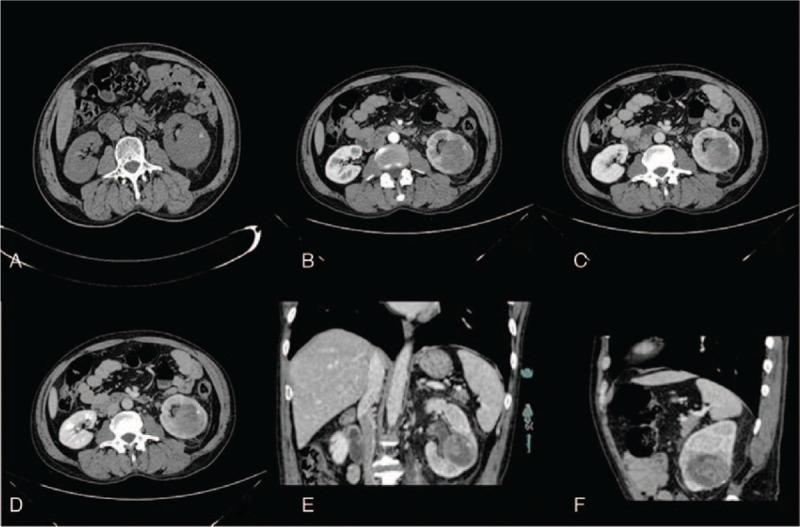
CT imaging. Axial plain CT scan (A) showed space-occupying lesions in the left kidney and about 4.5 cm × 4.0 cm in size. CT value is 25 to 35 Hu with calcification. The boundary of the lesion looks unclear. Axial enhanced CT (Figure B–D) are cortical phase, medullary phase, and excretory phase in order. The enhancement of the lesion was not obvious. A relatively low-density area is visible within the lesion. Coronal and anomalous images of the medullary phase of lesions are displayed in E and F. CT = computed tomography.
